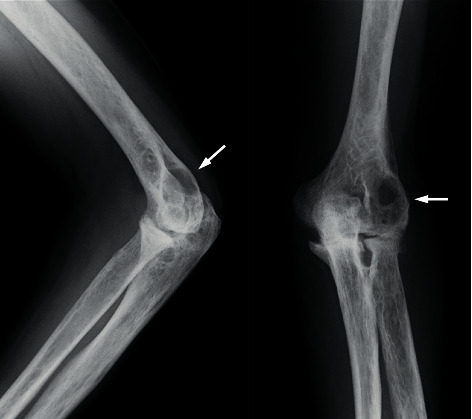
Plain radiograph of the left elbow at 10 months since the administration of subcutaneous tocilizumab injection. The transparent area gradually decreased, and bone formation progressed from the peripheral area (arrow). The size of evident geode is 10.8 mm × 8.3 mm.
